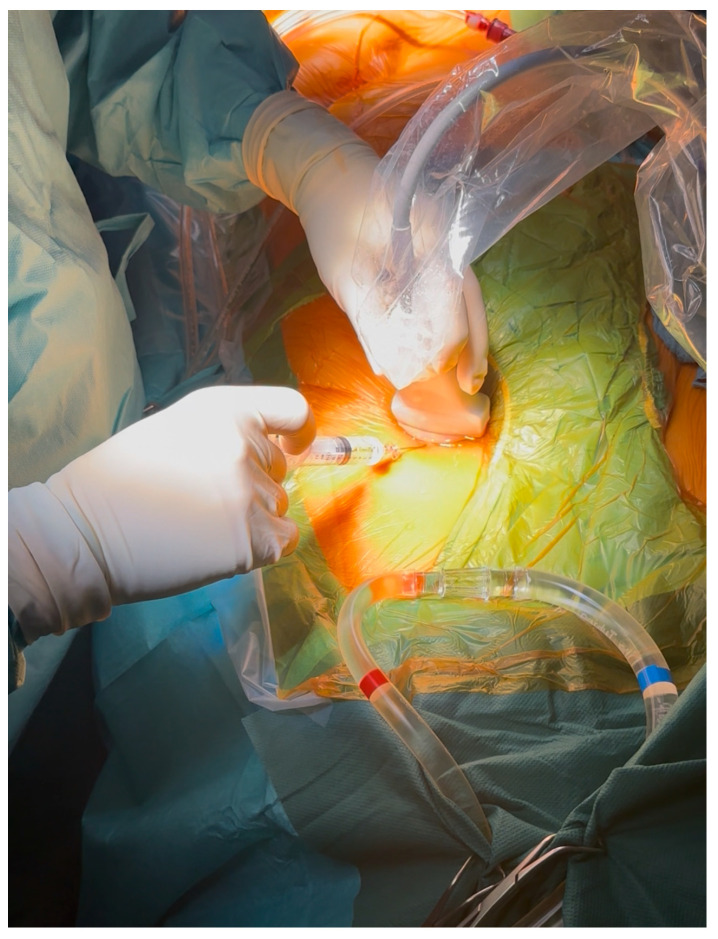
Ultrasound-guided percutaneous cannulation of the right femoral artery and vein.
